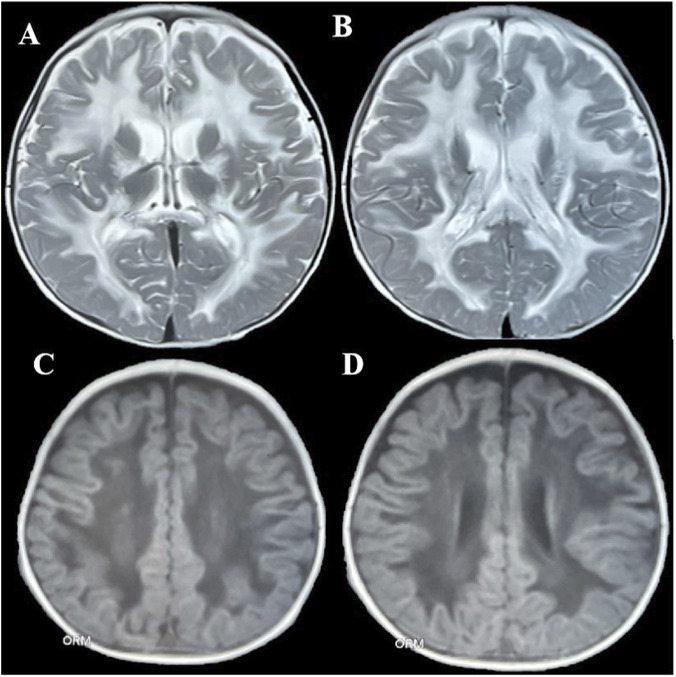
Brain MRI of the patients. (A,B) T2-weighted image of proband; (C,D) T1-weighted.
